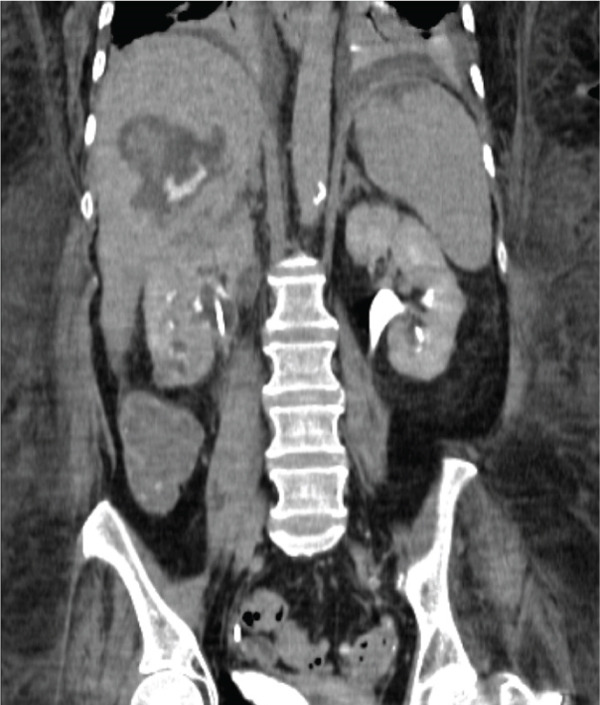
Computed tomography (CT) urogram performed 20 days after original presentation shows contrast extravasating from the right kidney and reaching the right hepatic lobe, confirming a pyelo‐hepatic fistulous tract.
